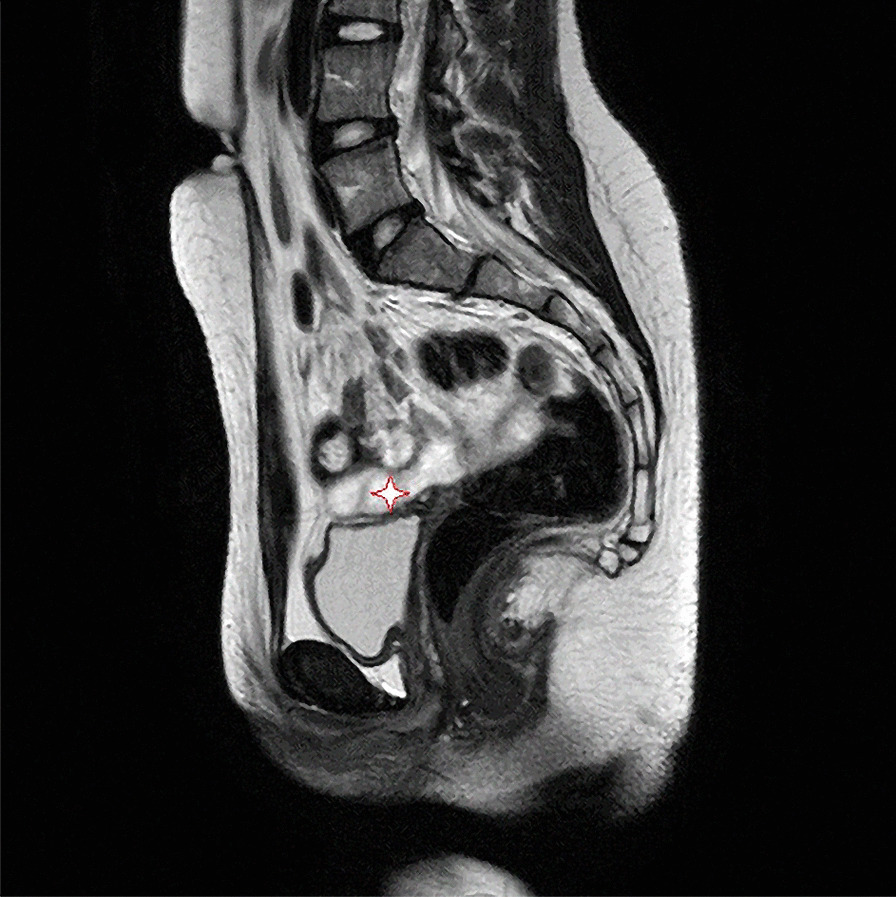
Nuclear magnetic resonance imaging. Medium sagittal image enhanced in T2. The absence of the uterus (star) is confirmed
